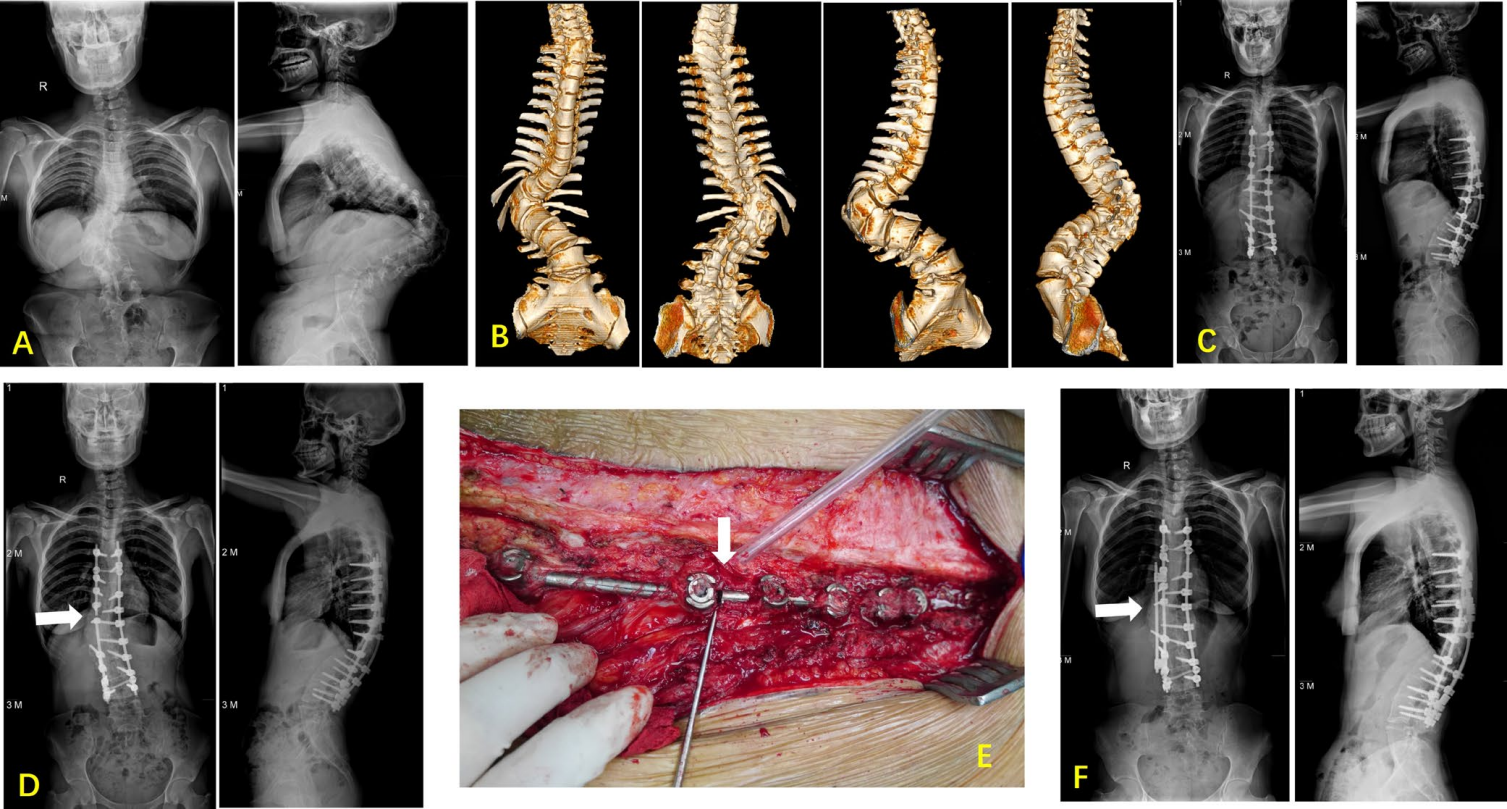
The patient was a 26‐year‐old female with congenital kyphoscoliosis. (A) Preoperative long‐standing scoliosis radiographs. (B) Preoperative three‐dimensional computed tomography scans. (C) Three‐column osteotomy and correction were performed, and good correction in coronal and sagittal plane deformity was obtained. (D) and (E) Postoperative and intraoperative photographs showed pseudarthrosis and implant failure during short term (20 months postoperatively), and an unplanned revision surgery was performed. (F) The full‐spine radiographs after unplanned revision, and the additional rod across the three‐column osteotomy were used.
